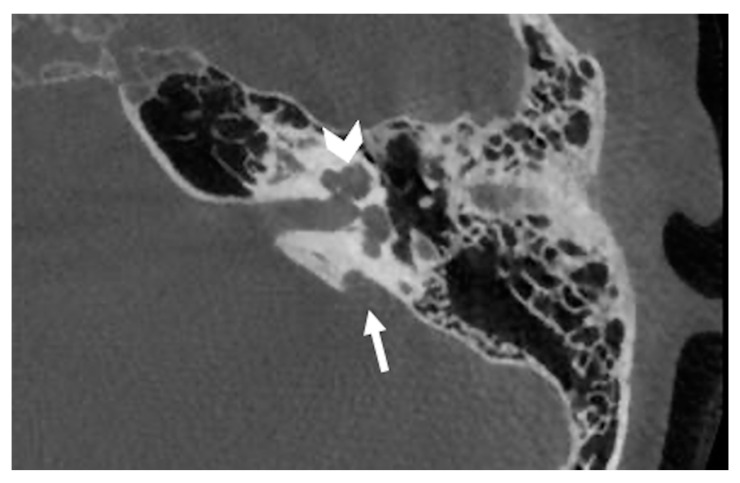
CT imaging of a left incomplete partition type 2. In this malformation the middle and apical turns appear to be fused (white arrowhead). Frequently, an enlarged vestibular aqueduct is also detectable (white arrow).
